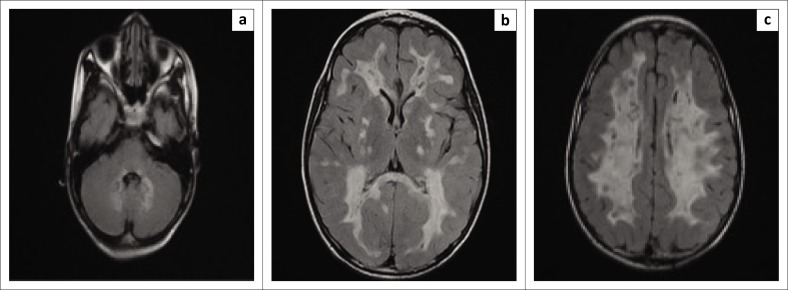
Initial magnetic resonance imaging (MRI) (after clinical presentation): (a) axial fluid attenuation inversion recovery (FLAIR) image with high signal in the cerebellar white matter tracts with associated mild cystic degeneration. (b and c) Axial FLAIR images show a classical MRI picture of CACH/VWM – diffuse symmetric signal abnormality with mild cystic degeneration in the cerebral white matter, sparing the U fibres. CACH/VWM, childhood ataxia and central nervous system hypomyelination (vanishing white matter disease).
